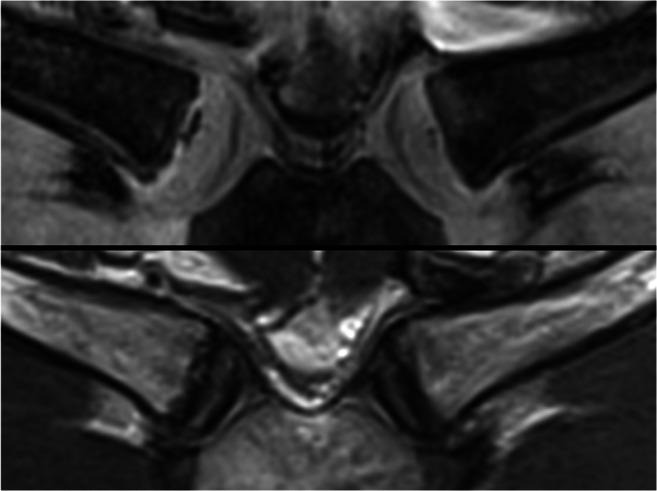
MR images of a 17.74-year-old male with stage 3a (right) and 2a (left) according to the enhanced Kellinghaus classification. Top: T1w 3D VIBE. Bottom: T2w TSE
